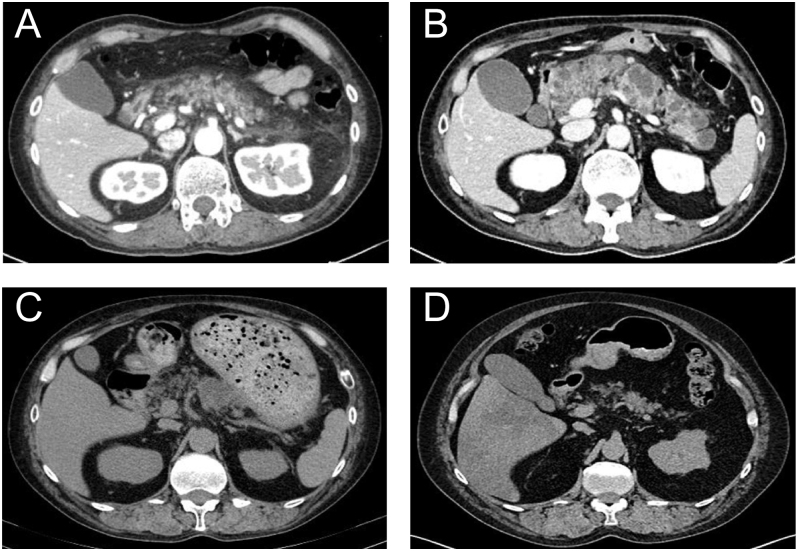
(A) Contrast-enhanced abdominal CT shows pancreatic enlargement and increased attenuation of the peripancreatic fat tissue. (B) Contrast-enhanced abdominal CT shows multiple cystic lesions in the pancreas. (C) Six months later, a non-contrast abdominal CT shows a reduction in the size of the pancreatic cystic lesion. (D) Eighteen months later, non-contrast abdominal CT shows complete resolution of the pancreatic cystic lesion.
